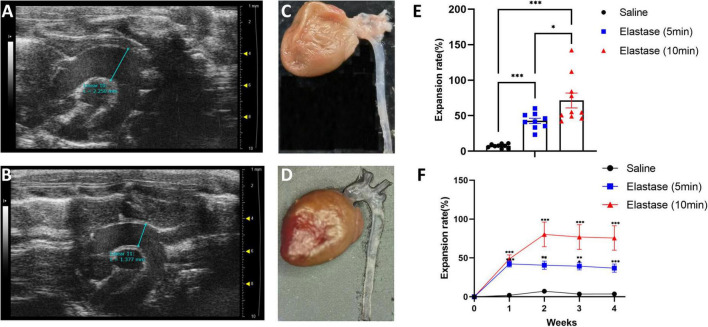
The ultrasonic image, morphology, and the expansion rate of proximal thoracic aortic aneurysm (PTAA) models. (A) The representative ultrasonic image of the PTAA (5-min elastase application), the maximal ascending aorta diameter is 2.25 mm 28 days after operation. (B) The representative ultrasonic image of the controlled thoracic aorta, the maximal ascending diameter is 1.38 mm 28 days after operation. (C) Representative morphology of PTAA. (D) Representative morphology of the controlled thoracic aorta. (E) Fourteen days after operation, the aortic dilation rate in 5-min, 10-min elastase, and saline application groups. The aortic dilation rate in different groups were compared with the two-tailed Mann–Whitney tests,P* < 0.05, ^*^*P* < 0.001. (F) Aortic dilation rate over time during PTAA formation. Maximal ascending aortic diameter (mean ± s.e.m.) in male C57BL/6J exposed to 5-min (*n* = 9) or 10-min (*n* = 9) elastase application or exposed to 10-min saline (*n* = 9) over time. The aortic dilation rate between the 5-min or 10-min elastase application group and the saline group were compared with the two-tailed Mann–Whitney tests, ^^*P* < 0.01, ^*^*P* < 0.001.
